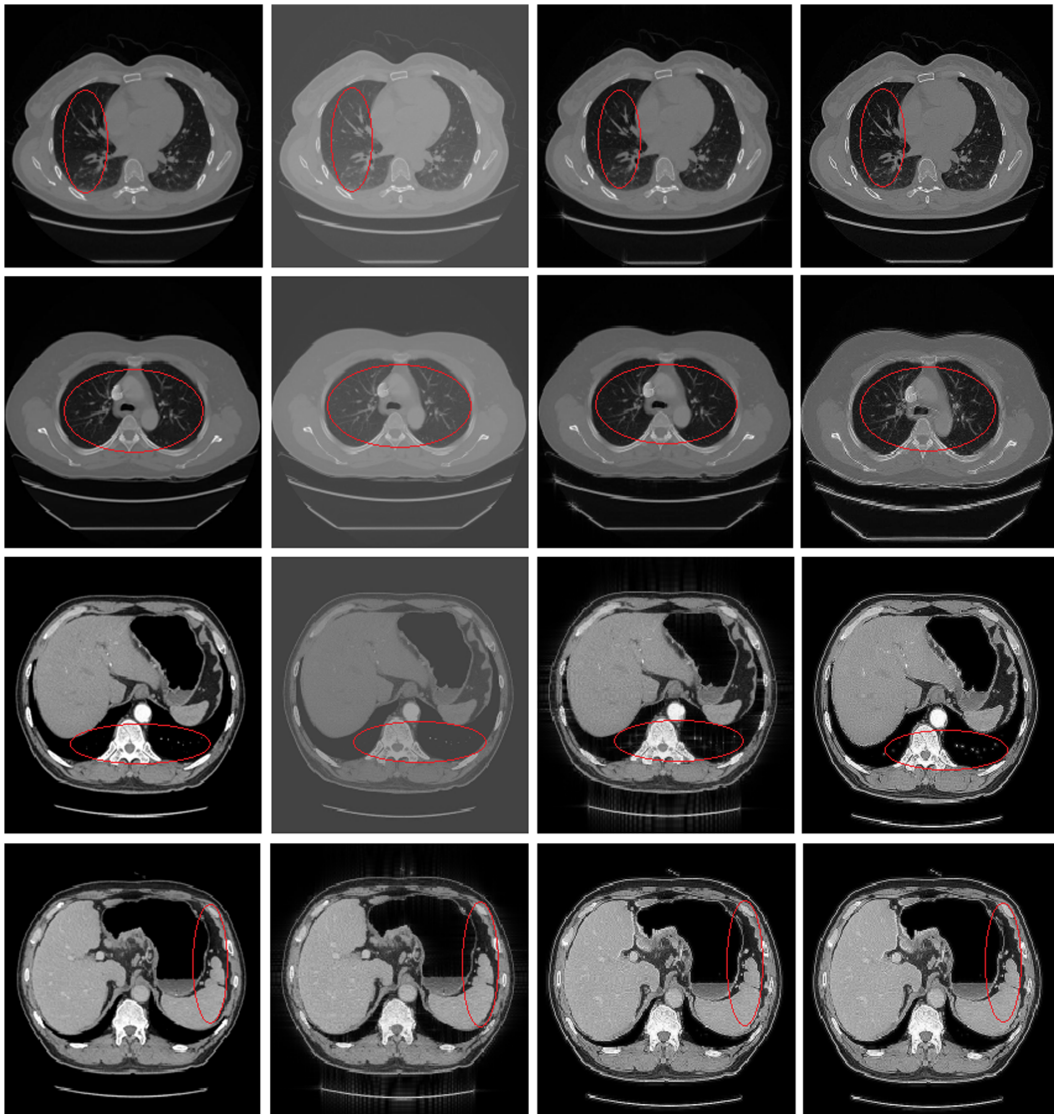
Shows comparisons of different methods. From top to bottom: chest CT experiment, lung CT experiment, stomach CT (1) experiment, stomach CT (2) experiment. From left to right in each row: the original image, the recovery image using Wang's method, the recovery image using Hussien's method, the recovery image using our method. It needs to say, we select the RPCA model in chest CT and lung CT, and select the GoDec model in stomach CT. We can see that using our method to restore the medical CT image sequence can obtain satisfactory results, the recovery images can show high contrast, clear detail information and clear organ boundaries which are especially shown in the components circled with red ellipses in the images.
